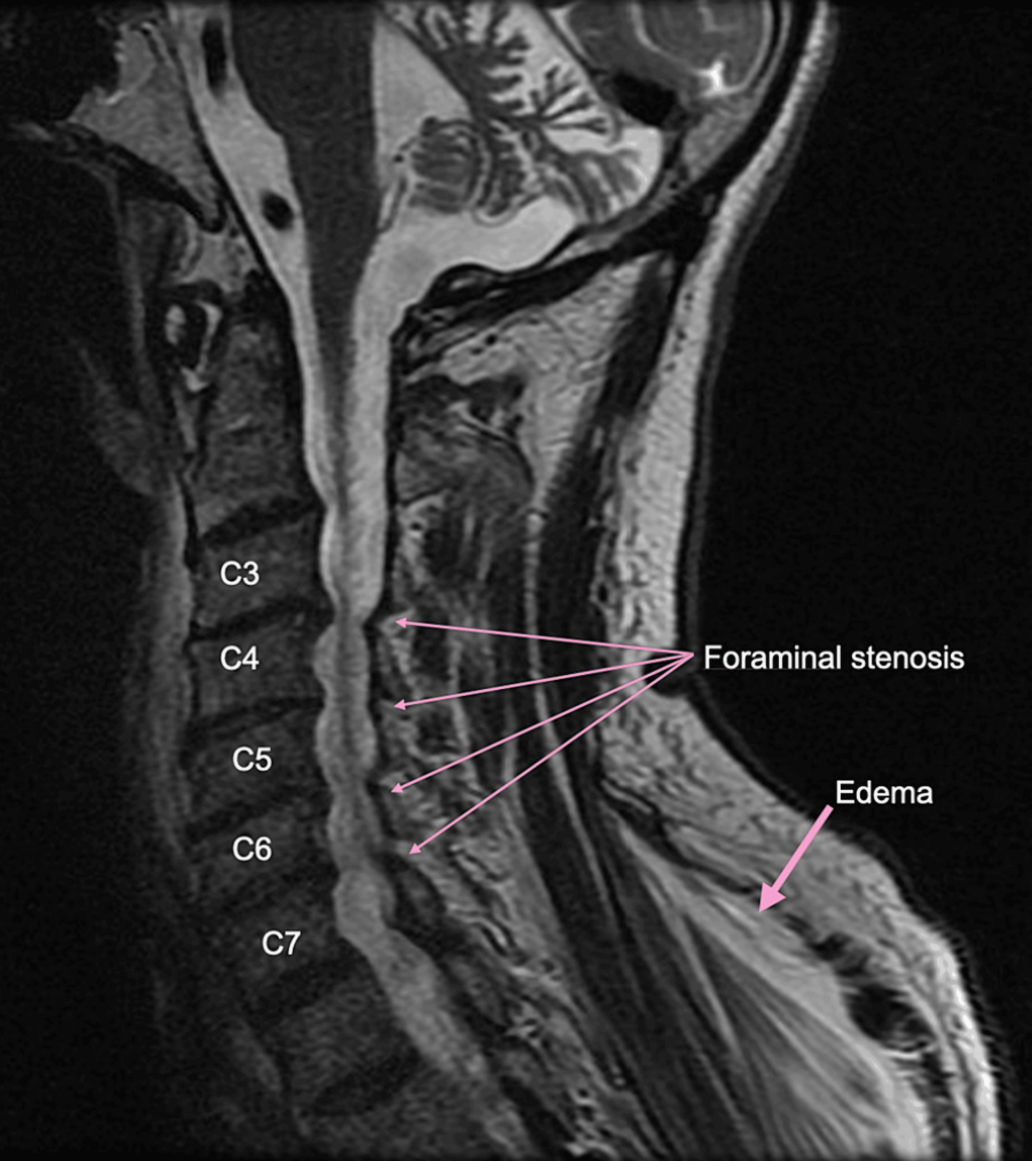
MRI cervical spine without contrast Spondylosis has resulted in multilevel mild spinal canal stenosis and moderate to severe neural foraminal stenosis from C3 to C7. There is no evidence of cord compression. Additionally, there is evidence of edema in the back and shoulder musculature, along with cerebellar volume loss.
